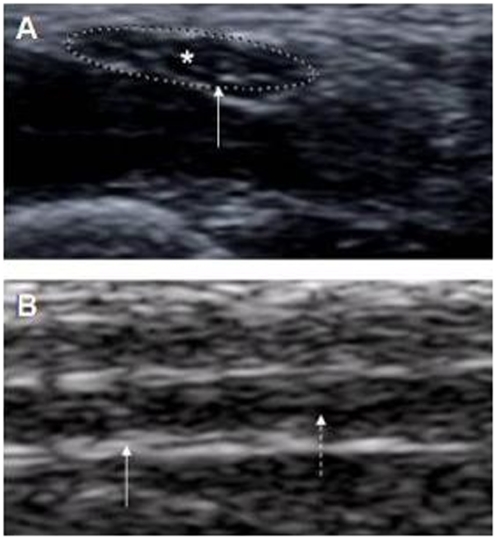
Ultrasonography and color Doppler images of peripheral nerves of healthy subjects. A Transverse scan of medial nerve from a healthy subject as denoted by dotted ellipse (CSA = 4.5 mm^2^) showing hypoechoic fascicles separated by hyperechoic areas in a ‘honeycomb’ like pattern with absence of blood flow signals; B longitudinal ultra sonogram of ulnar nerve from a healthy subject (arrows) with hyperechogenic bands in a linear pattern appearing as bundles of straw.
